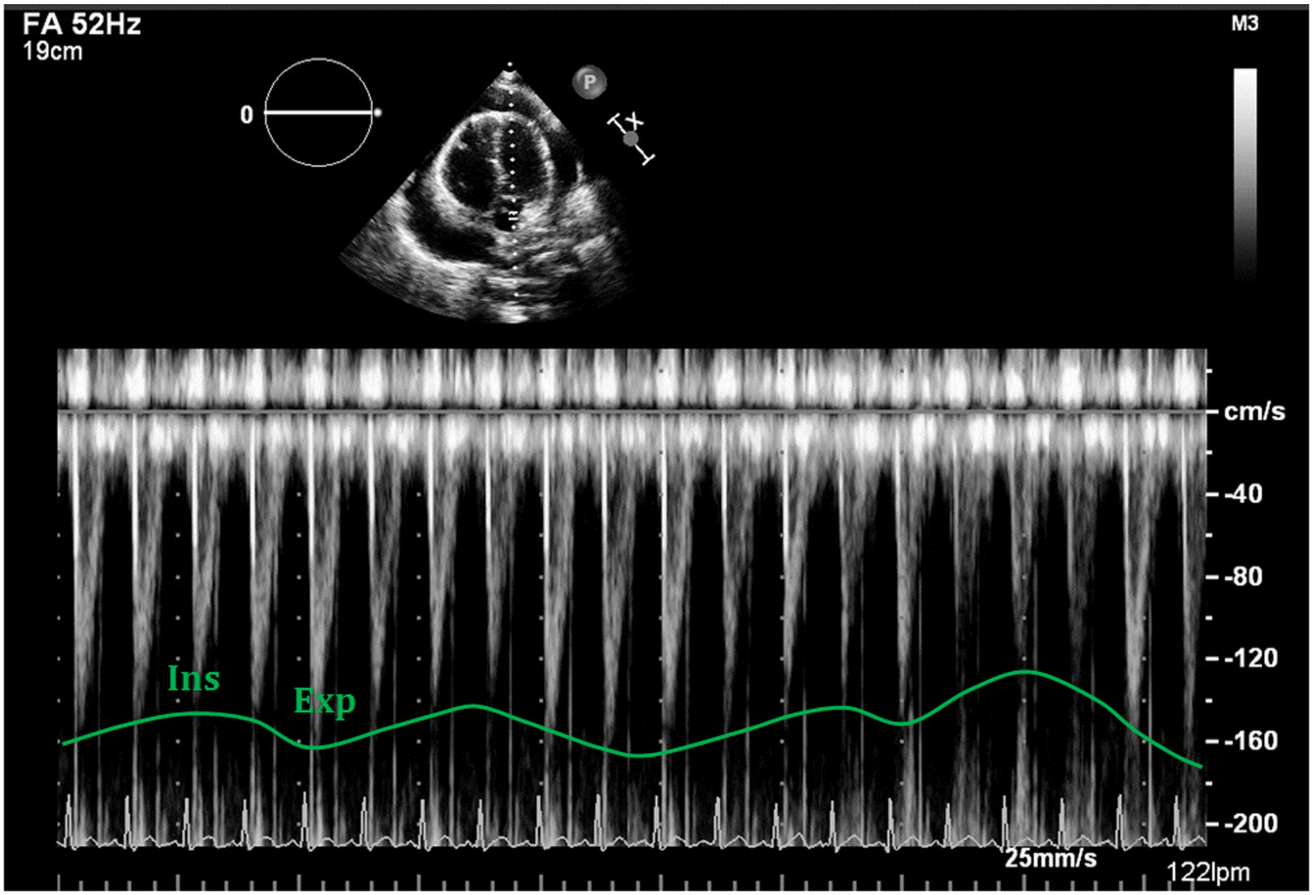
Respiratory variability seen with Doppler analysis of the left ventricular outflow tract, demonstrating decrease of >10% following deep inspiration.
